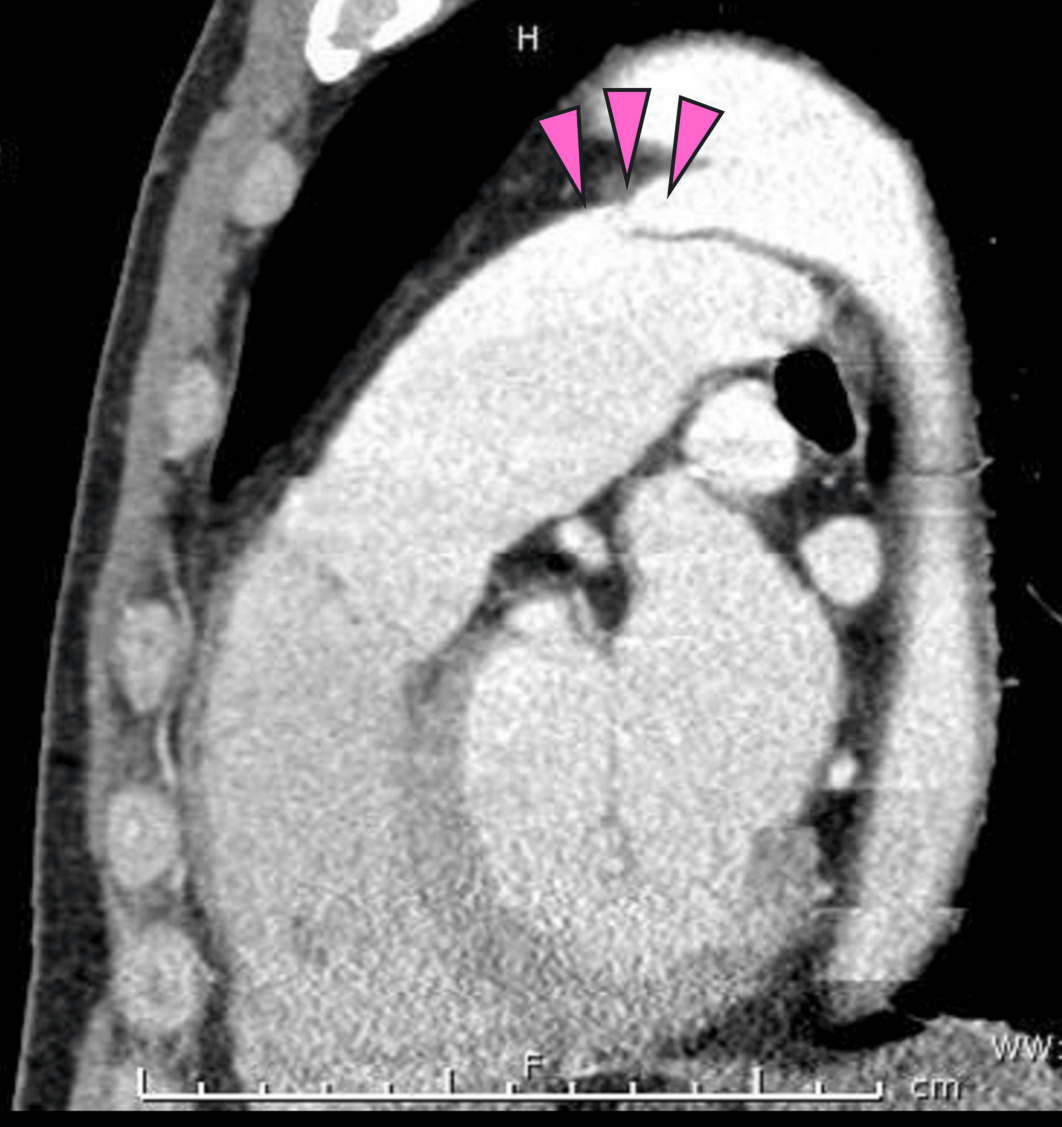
Contrast-enhanced computed tomography taken 17 years ago.
